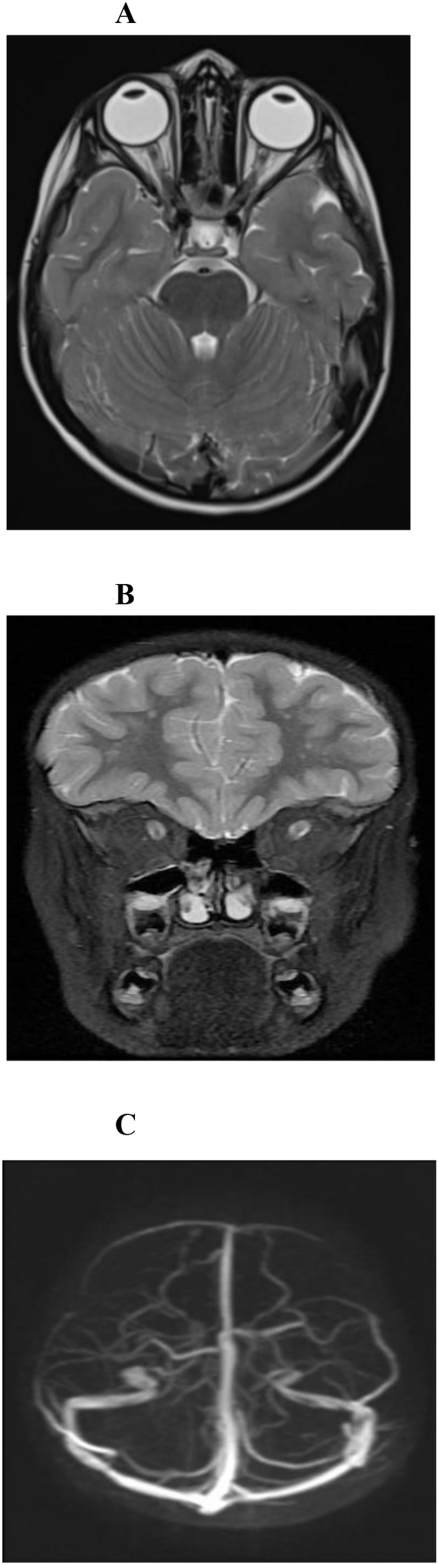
Follow-up neuroimaging after treatment. (A, B) Axial and coronal T2-weighted MRI of the brain and orbits at the level of optic nerve heads obtained after optic nerve sheath fenestration and lumbar puncture demonstrate a marked reduction in optic nerve sheath dilatation, with improvement in posterior scleral flattening, consistent with resolution of elevated intracranial pressure. Interval improvement is evident on the most recent imaging (B) compared with earlier post-treatment imaging (A, C) MR venography demonstrates partial recanalization of the left transverse sinus with improved opacification of the left sigmoid sinus and jugular bulb.
